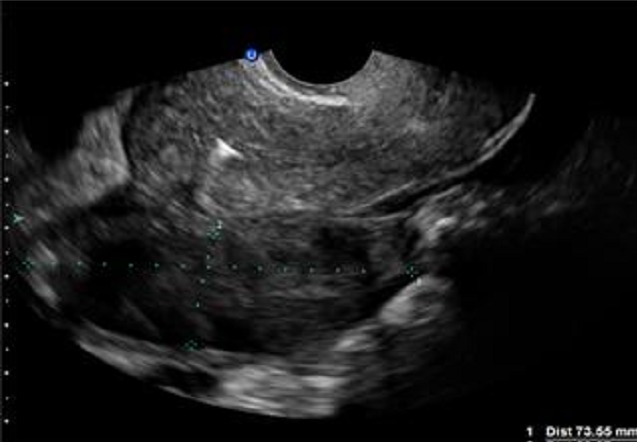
Échographie endovaginale montre une image rétroutèrine hétérogène, échogène tissulaire ne prenant pas le doppler couleur
